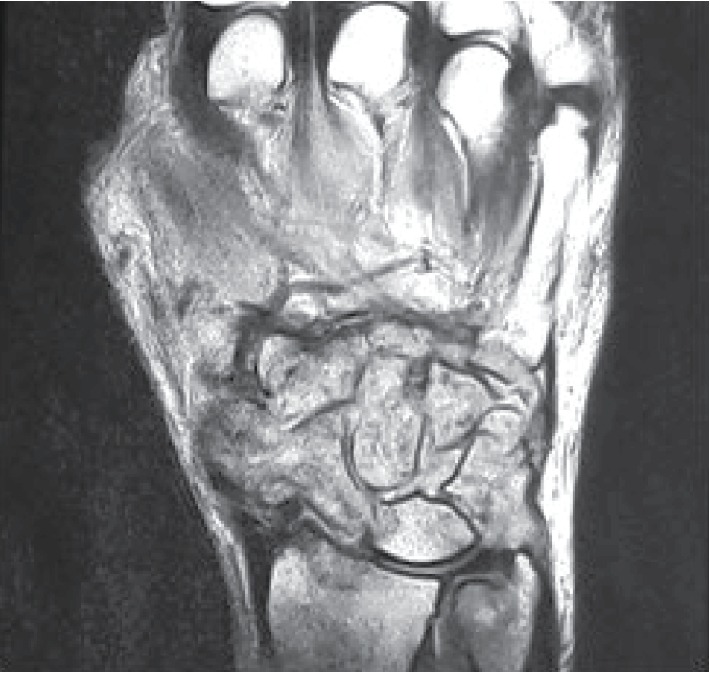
MRI image of the left hand showing destructive lesions in the carpal bones, surrounding focal fluid collections with narrowing of the intercarpal and carpometacarpal joints.
